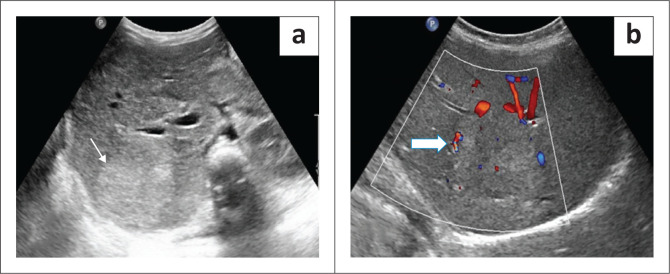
Focal nodular hyperplasia in a 5-year-old female who presented with abdominal pain. Transverse greyscale ultrasound (a) and colour Doppler (b) showing a well-circumscribed hyperechoic mass in the right lobe of the liver (thin arrow in a). The mass is showing mild vascularity on colour Doppler (thick white arrow).
